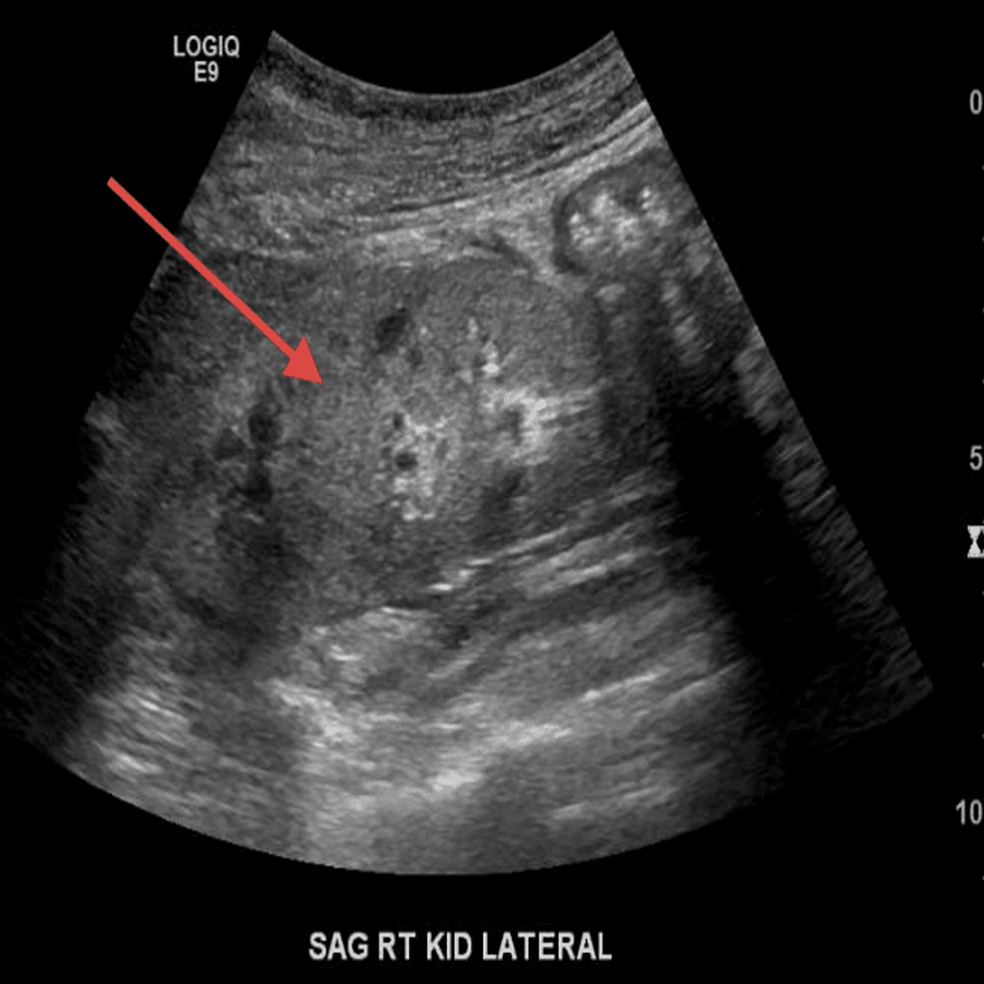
Ultrasound image of the right kidney Ultrasound of the right kidney showing echogenic parenchyma (red arrow).
